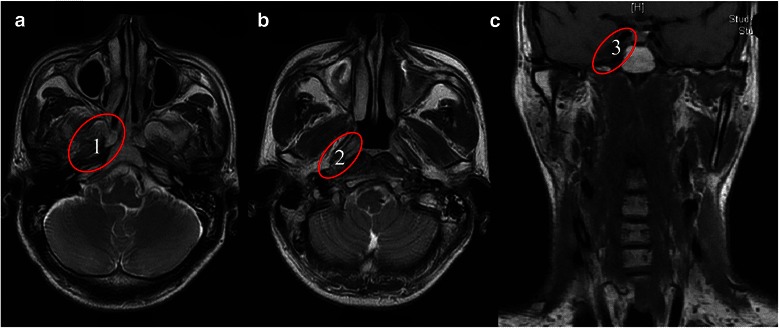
Magnetic resonance imaging findings of the common tumor invasion sites in patients with nasopharyngeal carcinoma (NPC). a, the skull base, the most common tumor invasion site (70.4 %); b, the parapharyngeal space (49.8 %); c, the intracranial cavity (32.3 %). The numbers in the pictures represent the order of incidence of tumor invasion
